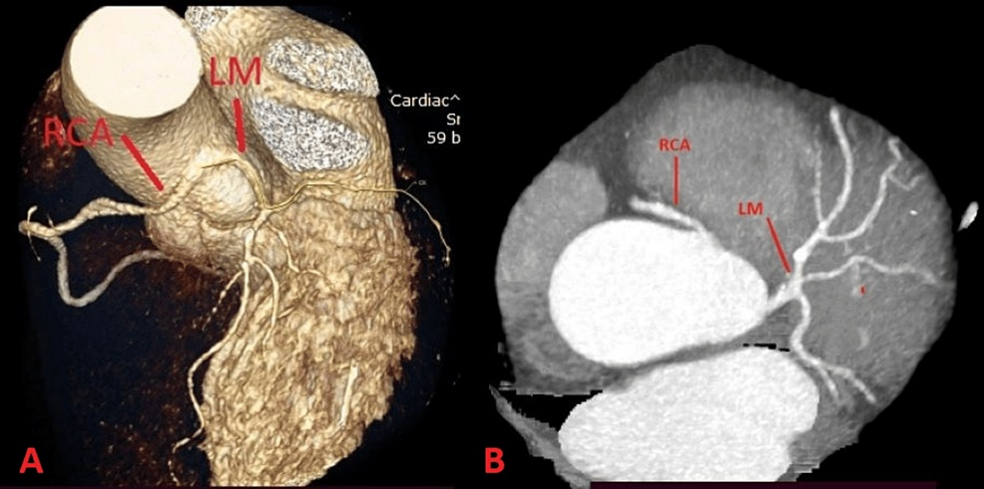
Case 4 A) Three-dimensional CCTA image showing LM and RCA originating from the sino-tubular junction above the left coronary sinus. B) Two-dimensional CCTA image showing LM originating from the sino-tubular junction and RCA with an interarterial course between the aortic bulb and the pulmonary trunk. CCTA: Coronary CT Angiogram; RCA: Right Coronary Artery; LM: Left Main
